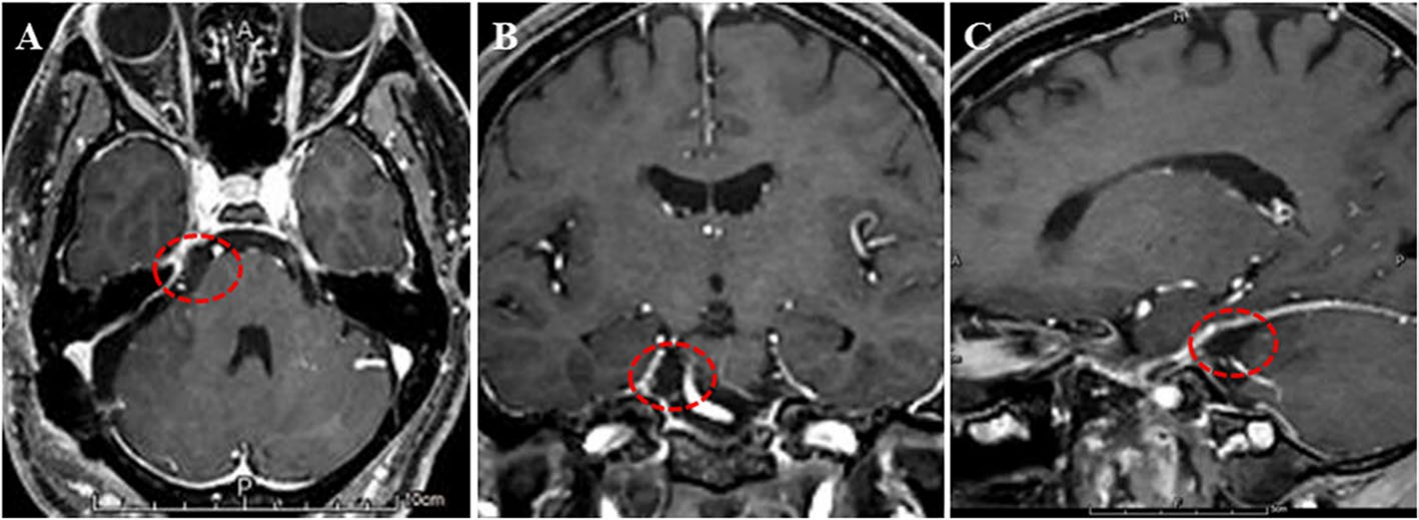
Postoperative MRI images of case 2. A Postoperative axial (A), coronal (B), and sagittal (C) enhanced head MRI of the patient in case 2 showed that the meningioma was totally removed
